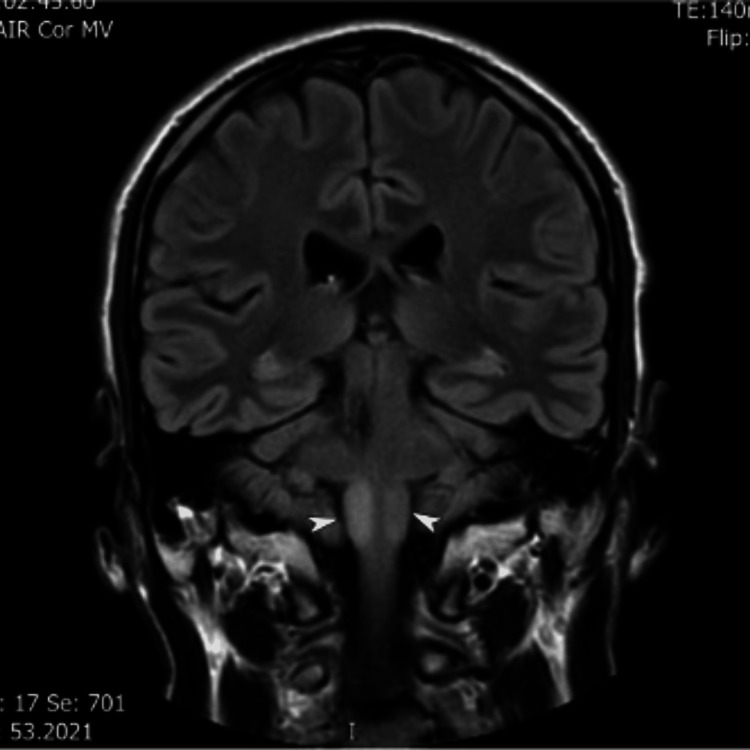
Coronal FLAIR (Fluid Attenuated Inversion and Recovery) MRI (six months after presentation) showing bilateral hypertrophic degeneration of olivary nuclei, more conspicuous on the right
